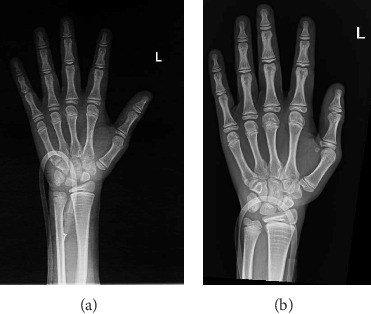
Hand radiographs for bone age assessment: (a) at 7 years 11 months chronological age, (b) at 9 years 3 months chronological age.
